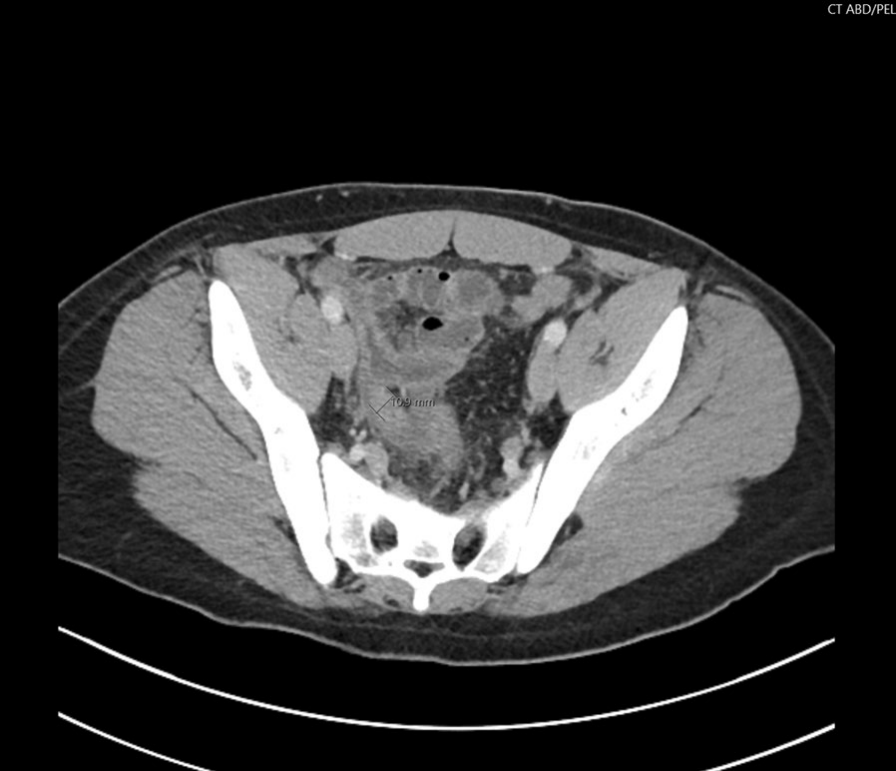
Transverse view of the abdominal CT demonstrating 0.9-mm dilated appendix with periappendiceal fluid in the pelvis
